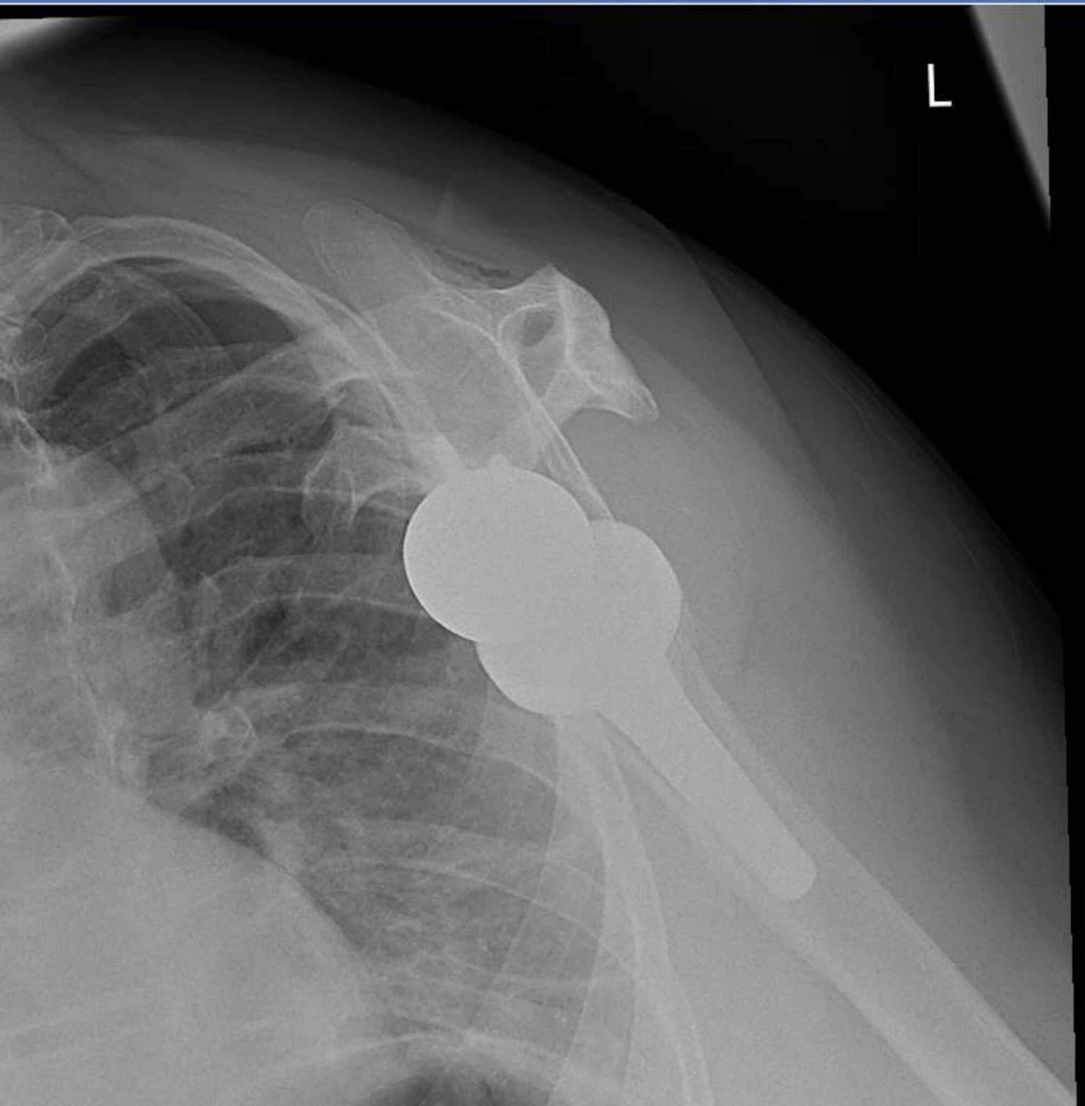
Initial post-operative plain radiograph of the left shoulder (Y-view)
